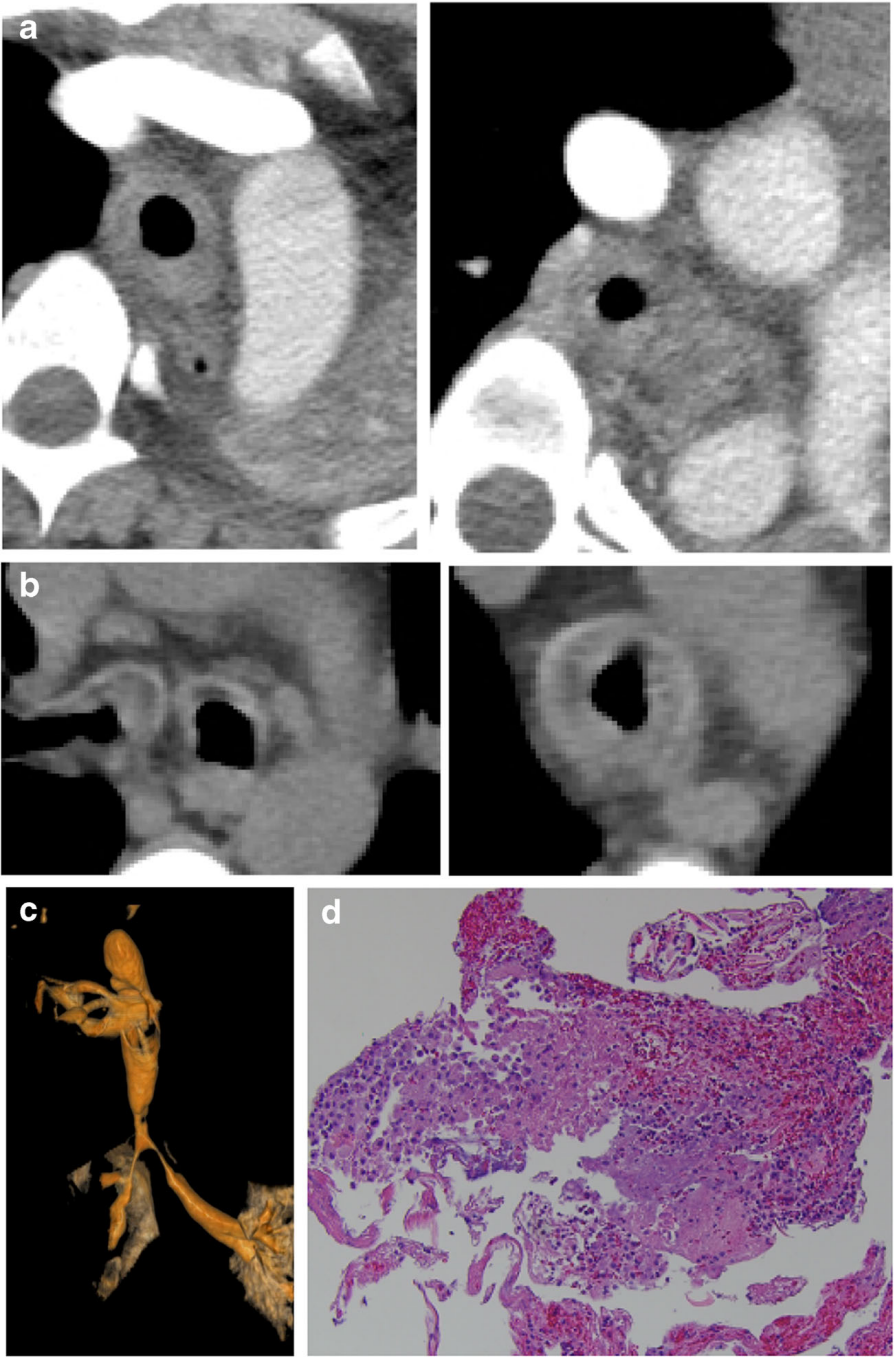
Twenty-five-year-old woman with airway stricture. a Contrast-enhanced axial CT and b non-contrast axial CT obtained 1 year later demonstrating non-enhancing focal narrowing of the distal trachea and proximal main stem bronchi. c 3D VR image. d Pathology reveals inflammatory exudate with fibrin and neutrophils compatible with epidermolysis bullosa
